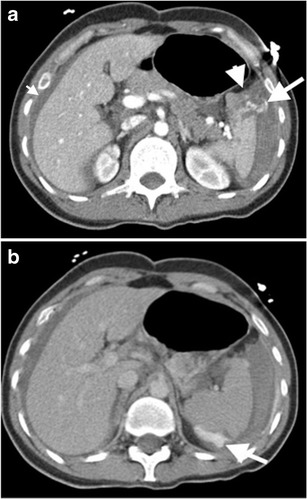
Active bleeding after splenic injury. Axial contrast-enhanced CT images at early (a) and delayed (b) phase demonstrate splenic laceration (*arrowhead in a*) and active intraperitoneal bleeding (*long arrows in a and b*). Absence of clot formation results from active massive haemorrhage, as clot formation is not fast as bleeding. Extravasated contrast material can accumulate in a dependent part of the body without restriction of any clotted hematoma as of yet (*arrow in b*). Moreover, the presence of perihepatic fluid (*short arrow in a *) supports the evidence of massive bleeding, since blood can act in a pattern similar to the free fluid, due to a decreased percentage of clot formation
